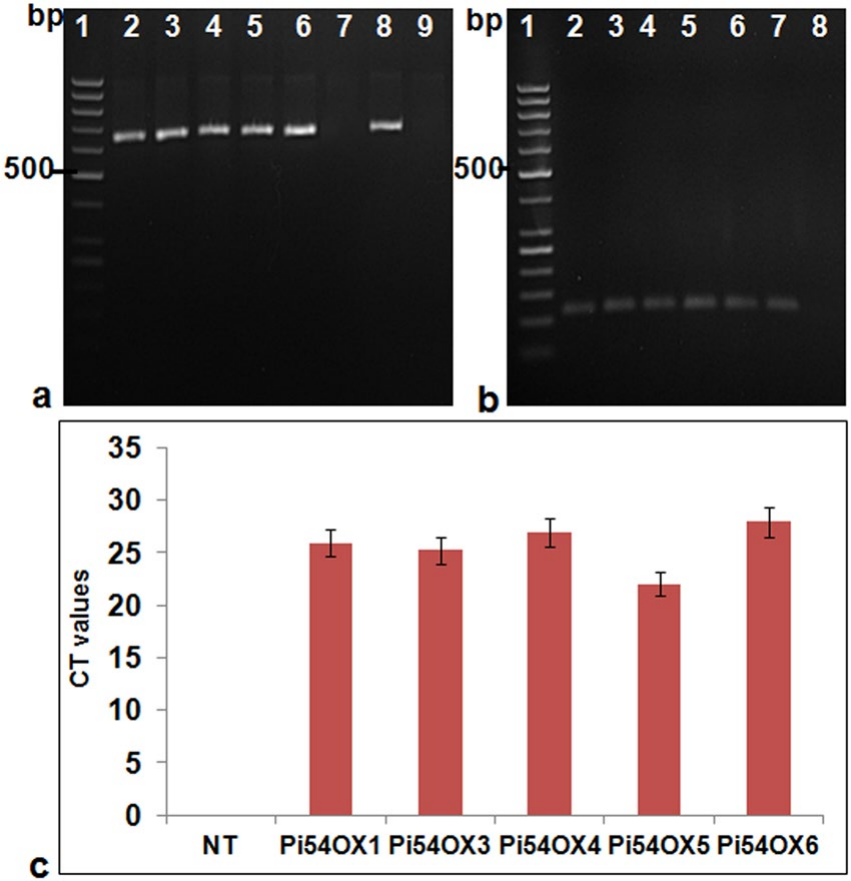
Reverse transcriptase PCR analysis of transgenic plants. (a) Using *GFP:Pi54* specific primers in transgenic Pi54OX and non-transgenic (NT)-control TP 309; Lane 1: 500 bp ladder, Lane 2–6: *Pi54OX* 1,3,4, 5 and 6, Lane 7: NT- control TP309; Lane 8: pRTV construct, Lane 9: No template control. (b) Internal control; Actin gene amplification in transgenicPi54OX and NT-control TP 309; Lane 1: 500 bp ladder, Lanes 2–6: Pi54OX 1,3,4 5 and 6, Lane 7: NT-control TP309, Lane 8: No template control. (c) CT values during qRT- PCR using *GFP::Pi54* specific primers in transgenic Pi54OX and non-transgenic (NT)-control TP 309; Lane 1: NT-control TP309; Lane 2–6: Pi54OX 1,3,4, 5 and 6.
